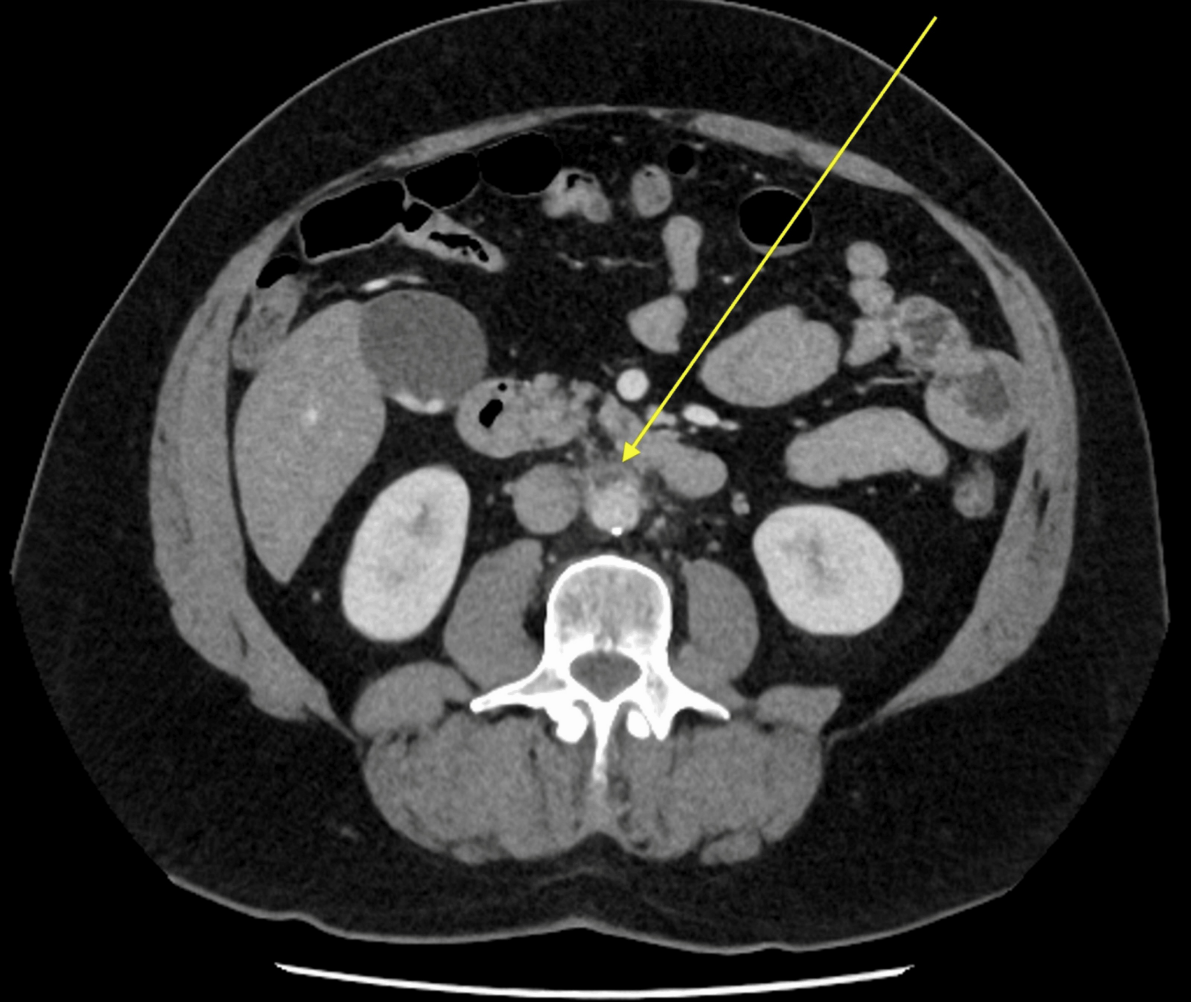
Axial contrast-enhanced CT of the abdomen and pelvis at the same level demonstrating fusiform dilatation and periaortic fat stranding, corresponding to early aortitis or atherosclerotic dilatation.
